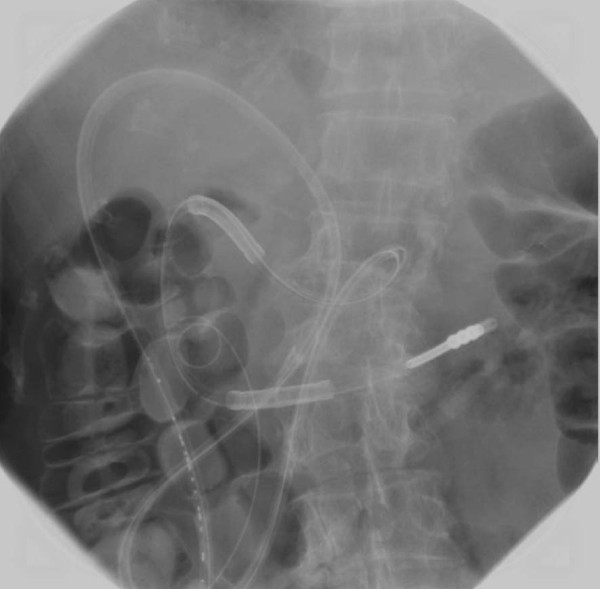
Abdominal radiograph taken 28 days after gastrojejunostomy tube insertion. Contrast medium administered via the tube reached the transverse colon. No obstruction or leakage was found. Intestinal distention was improved at this time.
